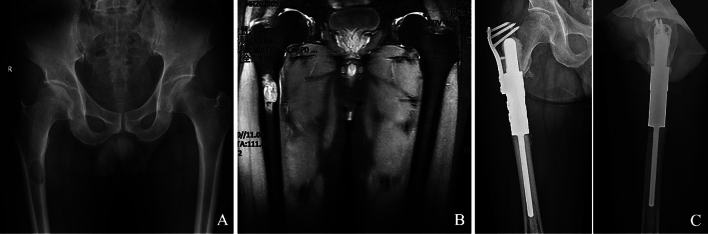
Cemented fixation of a proximal femoral prosthesis in a 58-year-old man following resection of a metastatic lung carcinoma. (A) Anteroposterior radiograph of the pelvis showing that there was an irregular low-density shadow below the greater trochanter of the right femur, and with obvious destruction of the bone cortex. (B) Fat-suppressed T2-weighted (T2W) image showing an irregular high-density shadow below the greater trochanter that with obvious destruction of the bone cortex of the right femur. (C) Anteroposterior and lateral radiographs showing that screws of the auxiliary lock plate were fixed through the greater trochanter.
